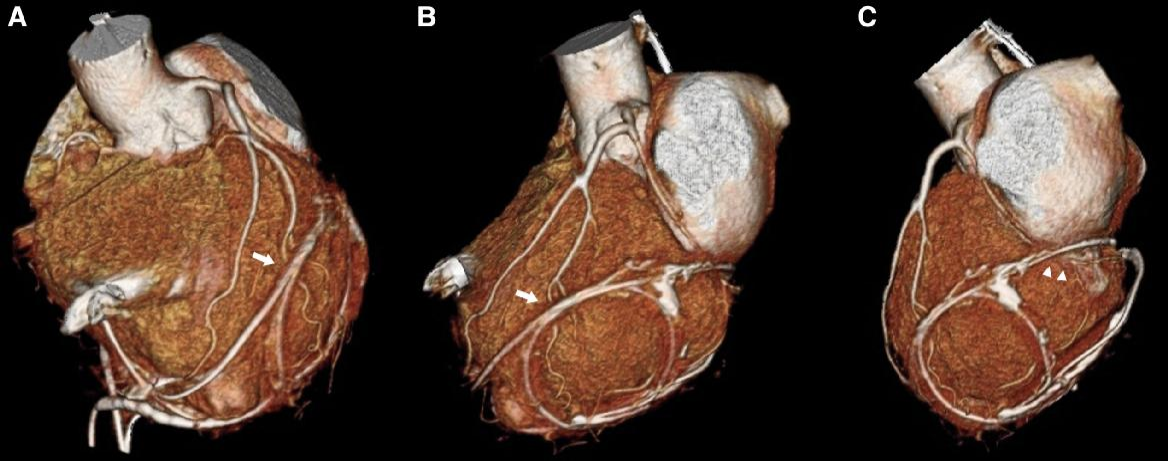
3D volume rendered coronary computed tomography angiography images clearly show multiple epicardial pacemaker leads constricting the apical and posterior-lateral free wall of the left ventricular, crossing the course of the distal first diagonal branch (arrows in *A* and *B*) and the mid-left-circumflex artery (arrowheads in *C*).
